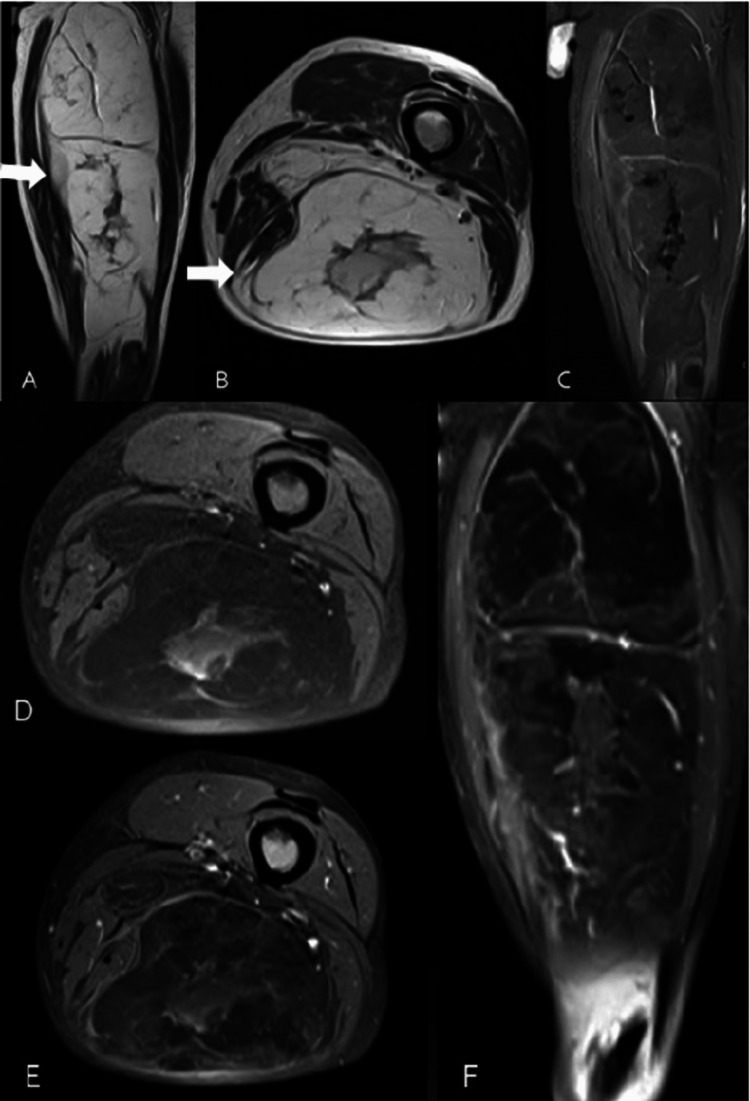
MRI of the thigh showing liposarcoma MRI of the left thigh in a 66-year-old male with gradually increasing thigh swelling that shows a well-defined capsulated large soft tissue lesion localized in the intramuscular plane of the posterior aspect of the thigh appearing hyperintense on T1-weighted (A) and T2-weighted images (B) with signal suppression on short tau inversion recovery (STIR) (C) images. The lesion shows heterogeneous post-contrast enhancement with the enhancement of the septae and soft tissue component on T1 fat-suppressed (T1FS) images (D-F). Diagnosis: liposarcoma.
